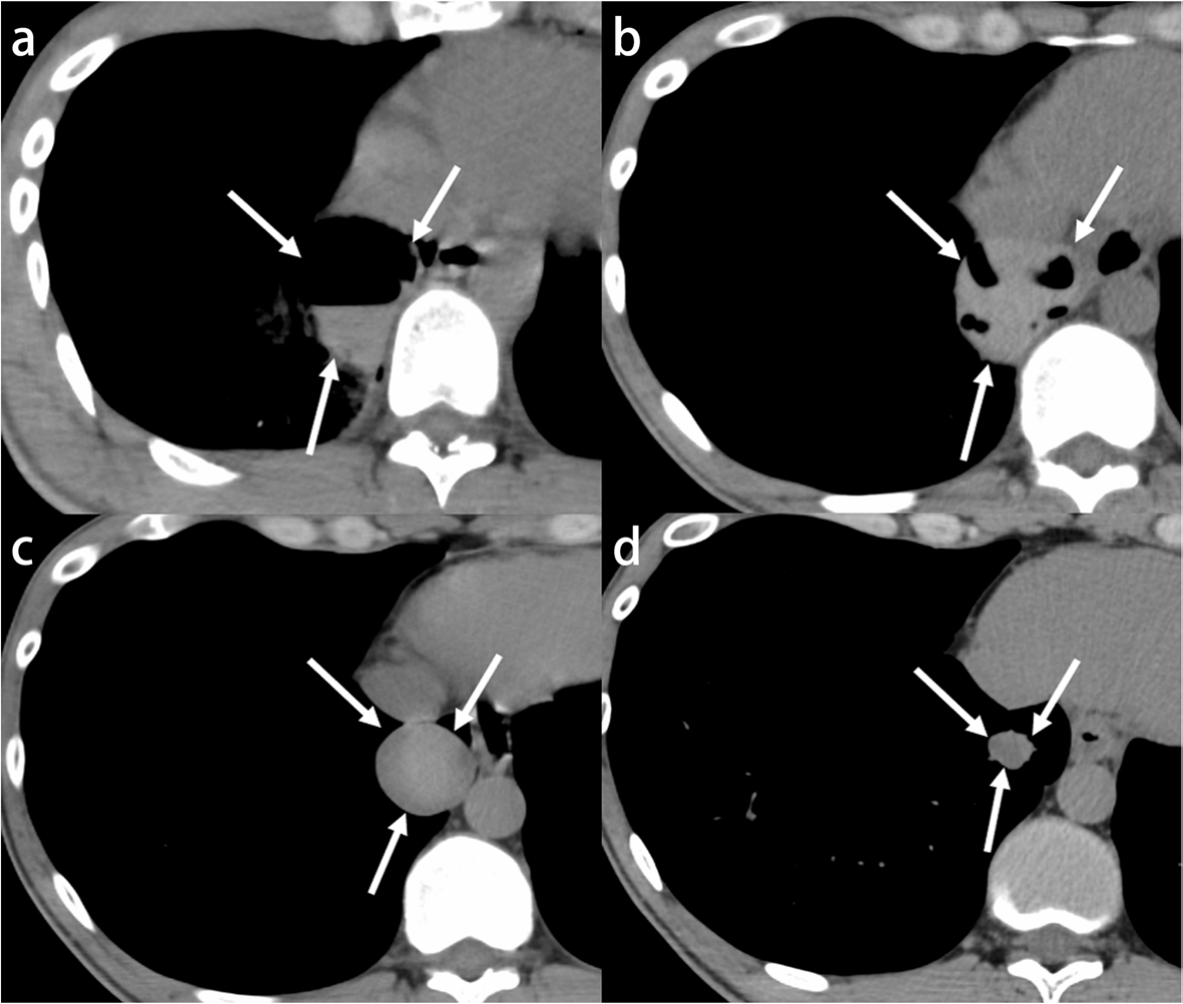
Pulmonary laceration due to traumatic injuries in a 28-year-old man. a CT image shows a high-attenuation air-fluid level in the right lower lobe (arrows). b The lacerated cyst has decreased in size, and the air bubble has been absorbed (arrows). c Although the air bubble has disappeared, the attenuation of the nodule remains high (arrows). d Attenuation of the nodule has decreased, and subsequent shrinkage of the nodule is shown (arrows)
